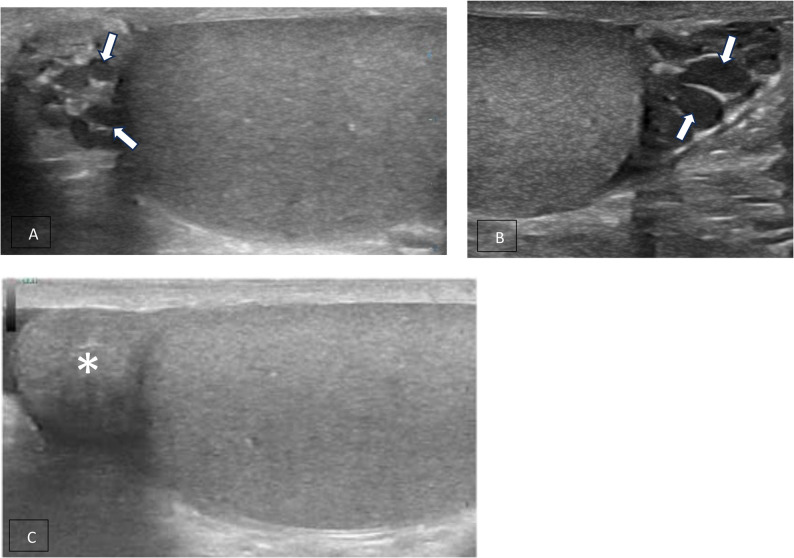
Scrotal point-of-care ultrasonography findings suggestive of obstructive azoospermia. (A, B) Scrotal POCUS images demonstrating multiple anechoic epididymal cysts (arrows) within the epididymal head, interpreted as secondary sonographic signs suggestive of obstruction. (C) Normal appearance of the epididymal head (asterisk) shown for comparison. These findings were interpreted in conjunction with the clinical history, physical examination, and hormonal profile
